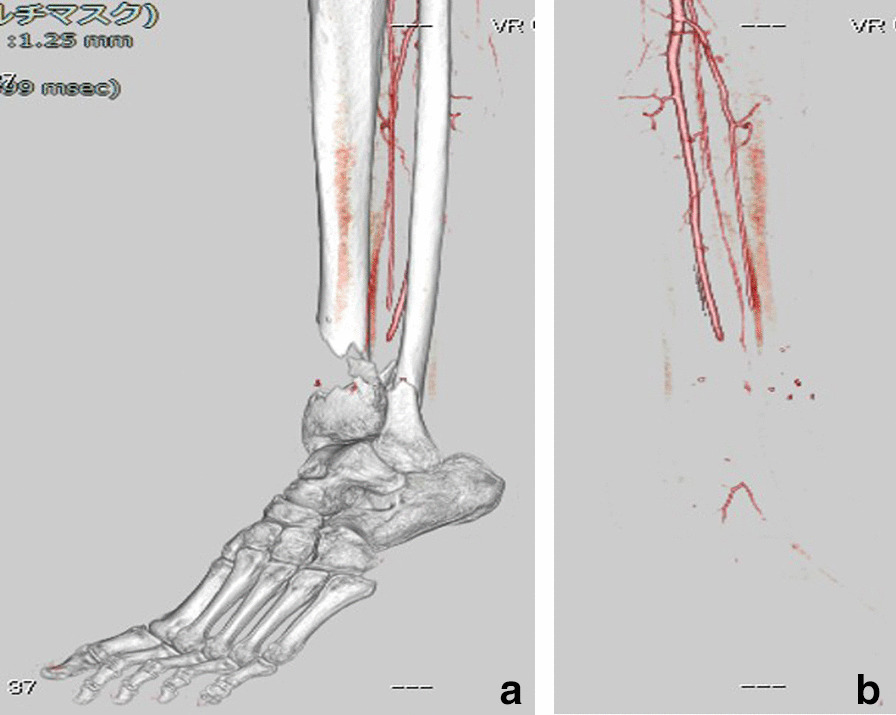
a 3D computed tomography angiography image with the bone; b 3D computed tomography angiography image without the bone *3D* three-dimensional
